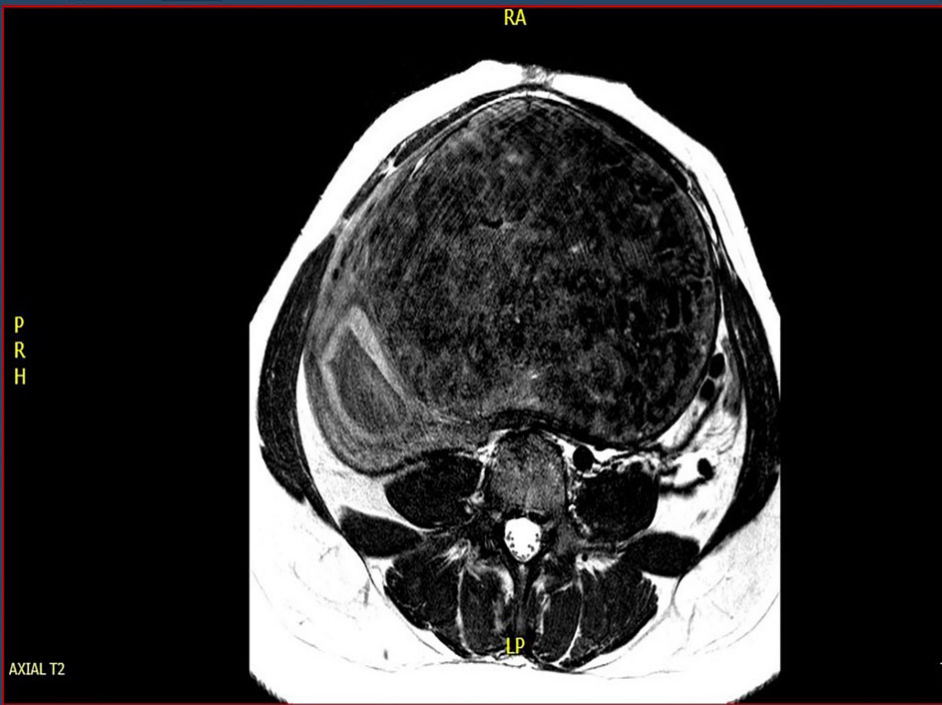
Transverse abdominal section on T2 magnetic resonance imaging showing right cervical deviation due to the mass growth.
